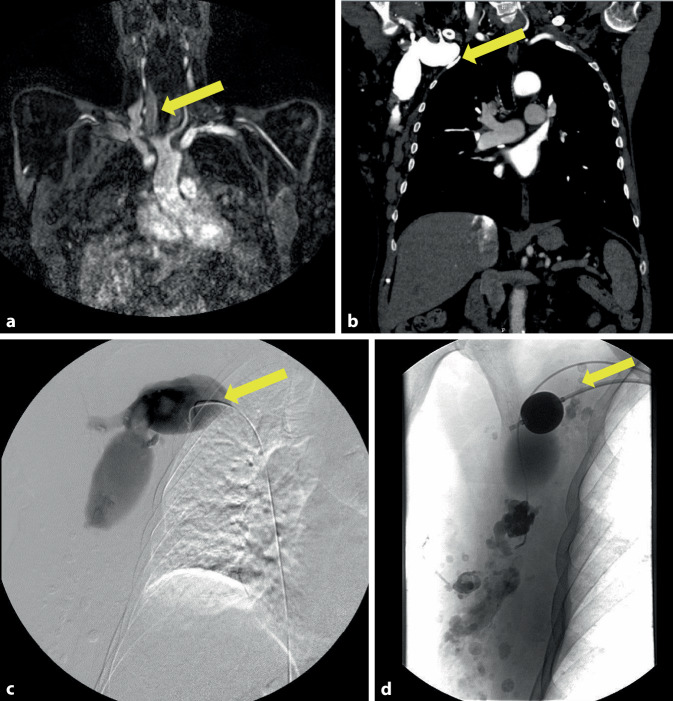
Fall 6: Aneurysmatische venöse Malformation mit Abfluss in eine ektatische V. subclavia rechts sowie ein venöser Abfluss über die V. azygos. a MR-Angiographie in koronarer Schichtung mit Darstellung des venösen Aneurysmas (*gelber Pfeil*), b CT-Angiographie des venösen Aneurysmakomplexes in koronarer Darstellung (*gelber Pfeil*), c Angiographische Darstellung des venösen Aneurysmas bei der Embolisation mit Embolisationskatheter (*gelber Pfeil*), d Embolisiertes venöses Aneurysma mit Blockungsballon (*gelber Pfeil*)
